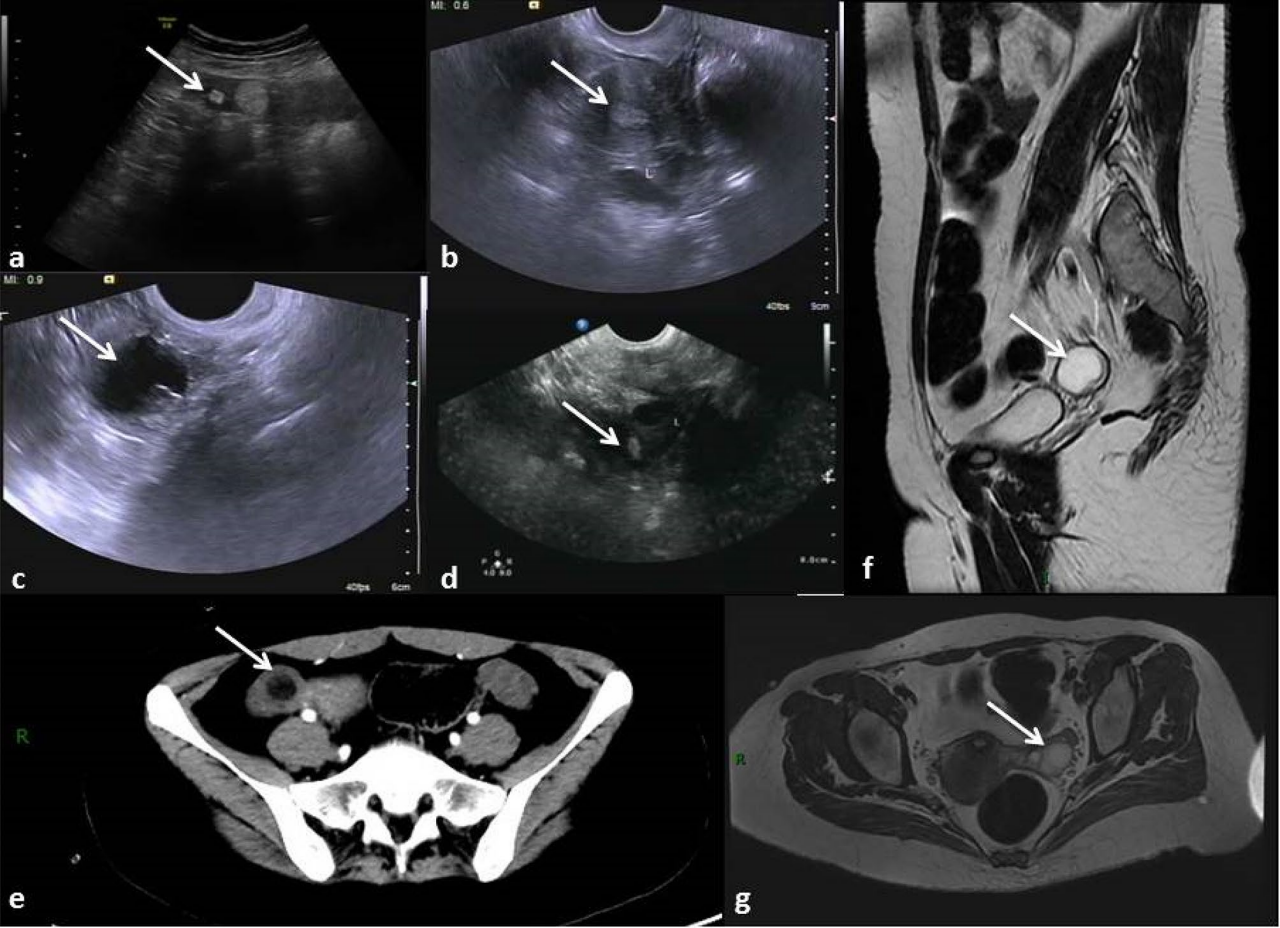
The ovarian cysts detected by ultrasound, CT and MRI for all six patients (arrows); a–d pictures of ultrasound in four patients (nos. 3–6); e CT scan for patient no. 2; f, g. cross-section and sagittal plane of pelvic MRI for patient no. 1
